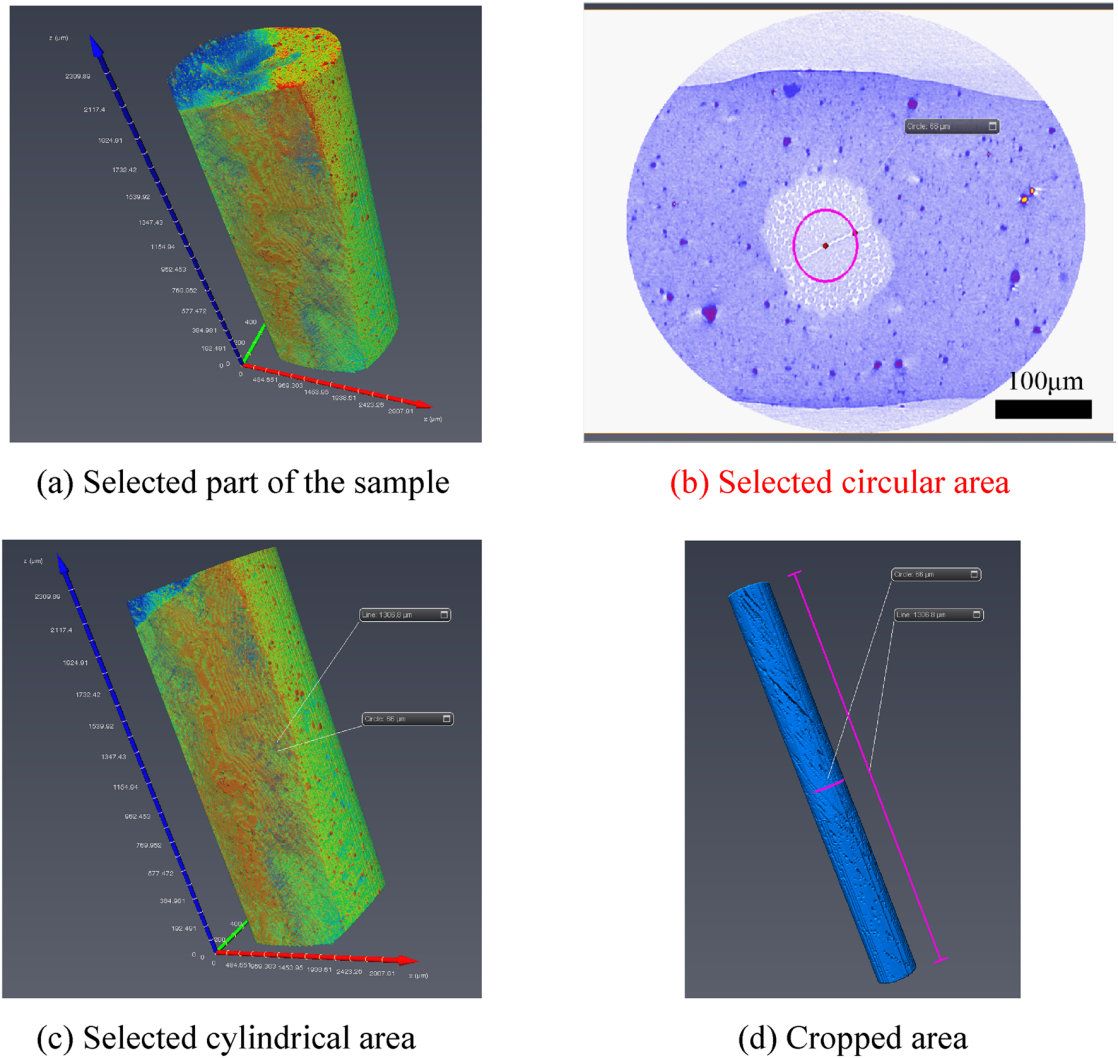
Area selection from the nylon-rubber CT images.
